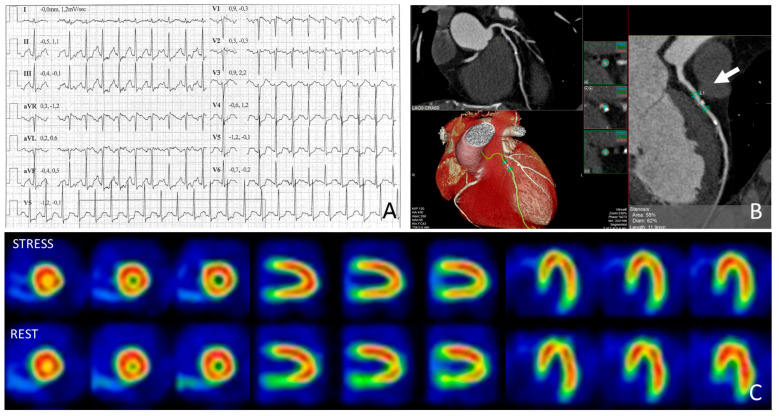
Representative example of an asymptomatic athlete with borderline ST-segment depression performed for preparticipation screening (A). Coronary computed tomography revealed moderate stenosis of the left anterior descending artery (B). However, stress-rest myocardial SPECT showed no inducible myocardial ischemia (C).
